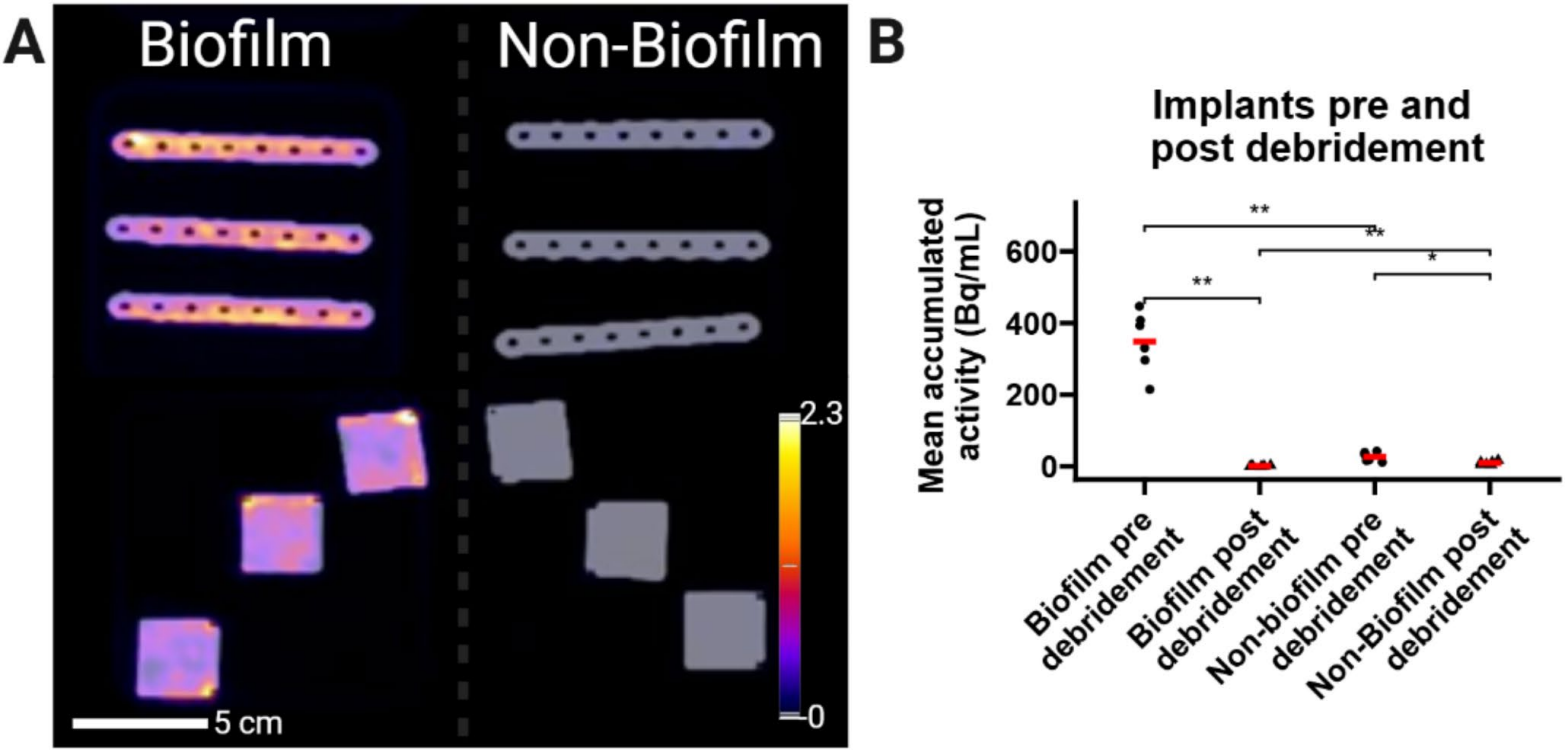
(A) PET/CT images showing the osteosynthesis plates used for the additional experiments. The images show accumulation of tracer on the plates that carry a bacterial biofilm. Values are expressed as kBq/mL. (B) Quantification of the in vitro data (mean accumulated activity in Bq/ml) validates the findings in the post-mortem experiment, showing that bacterial biofilms are effectively detected using the PET tracer, and that the effect of debridement can be visualized using this PET tracer in the presently applied human post-mortem implant infection model. *, *p* < 0.05. , *p* < 0.01
